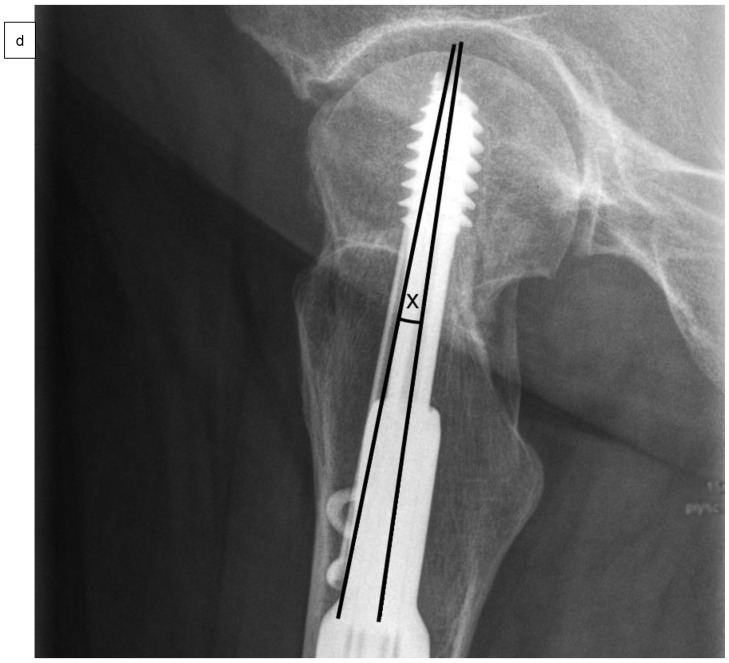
Measurement of the femoral neck angle (*) compared to the contralateral side (°), defined as the angle between the femoral neck axis and the bisecting line of the femoral shaft (a). The tip-apex distance (TAD) was defined as the calibrated summation of the distance between the tip of the SHS and the apex of the femoral head on anteroposterior and (not demonstrated) lateral radiographs (mm) (b), and the angle between SHS and ASR in the frontal plane (#) (c) and the axial plane (x) (d) by using biplanar conventional radiographs, six weeks postoperatively.
